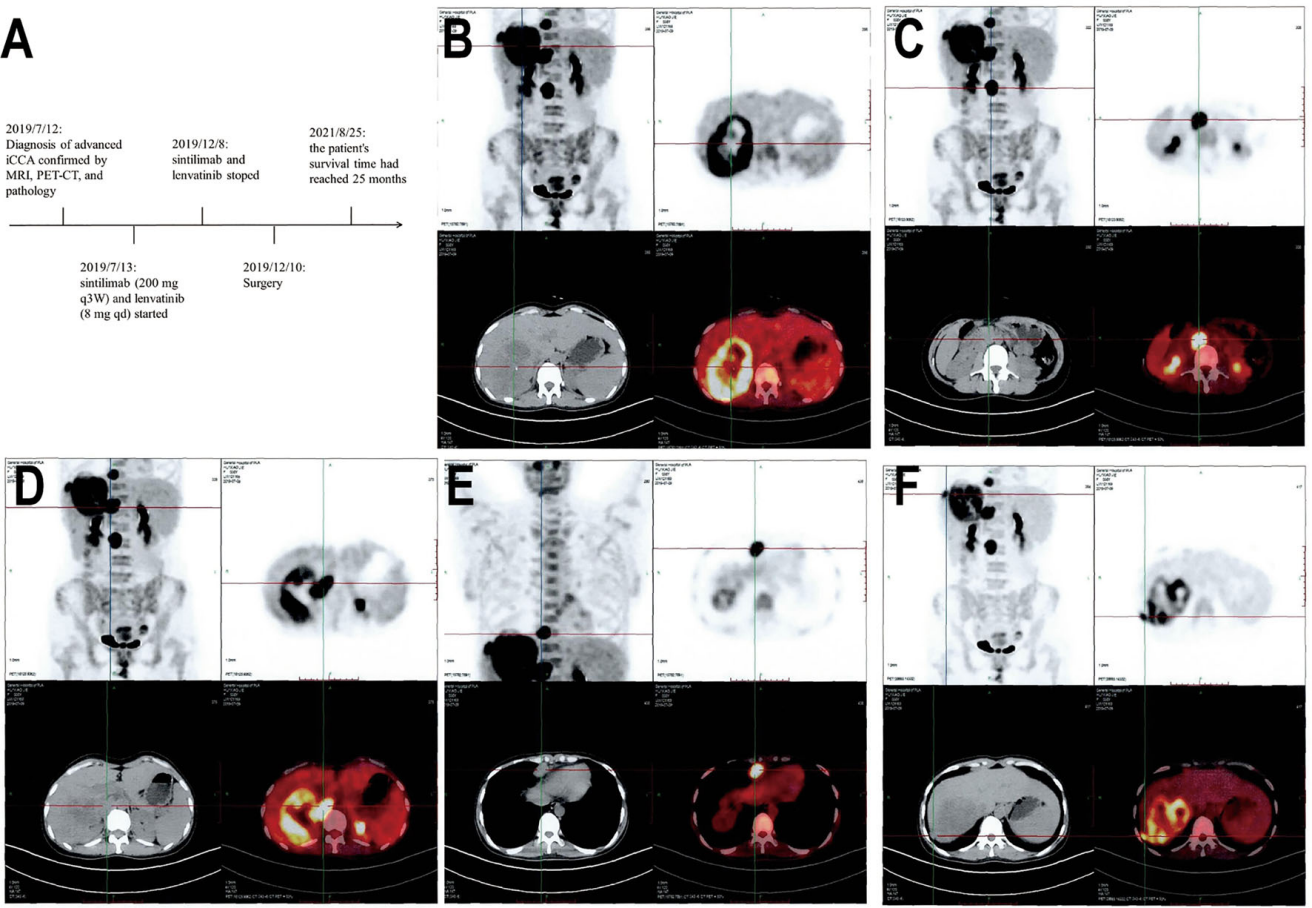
Timeline and PET-CT examination. (A) Indicates initial diagnosis, medication treatment, surgery, and follow-up timeline of the patient. (B) Shows a large mass in the right lobe of the liver with increased radioactive uptake of the solid component. (C) Shows multiple enlarged lymph nodes adjacent to the abdominal aorta with increased radioactive uptake. (D) Shows increased nodular radioactive uptake in the inferior vena cava. (E, F) Show anterior costal diaphragm nodule and right diaphragmatic nodule with increased radioactive uptake.
